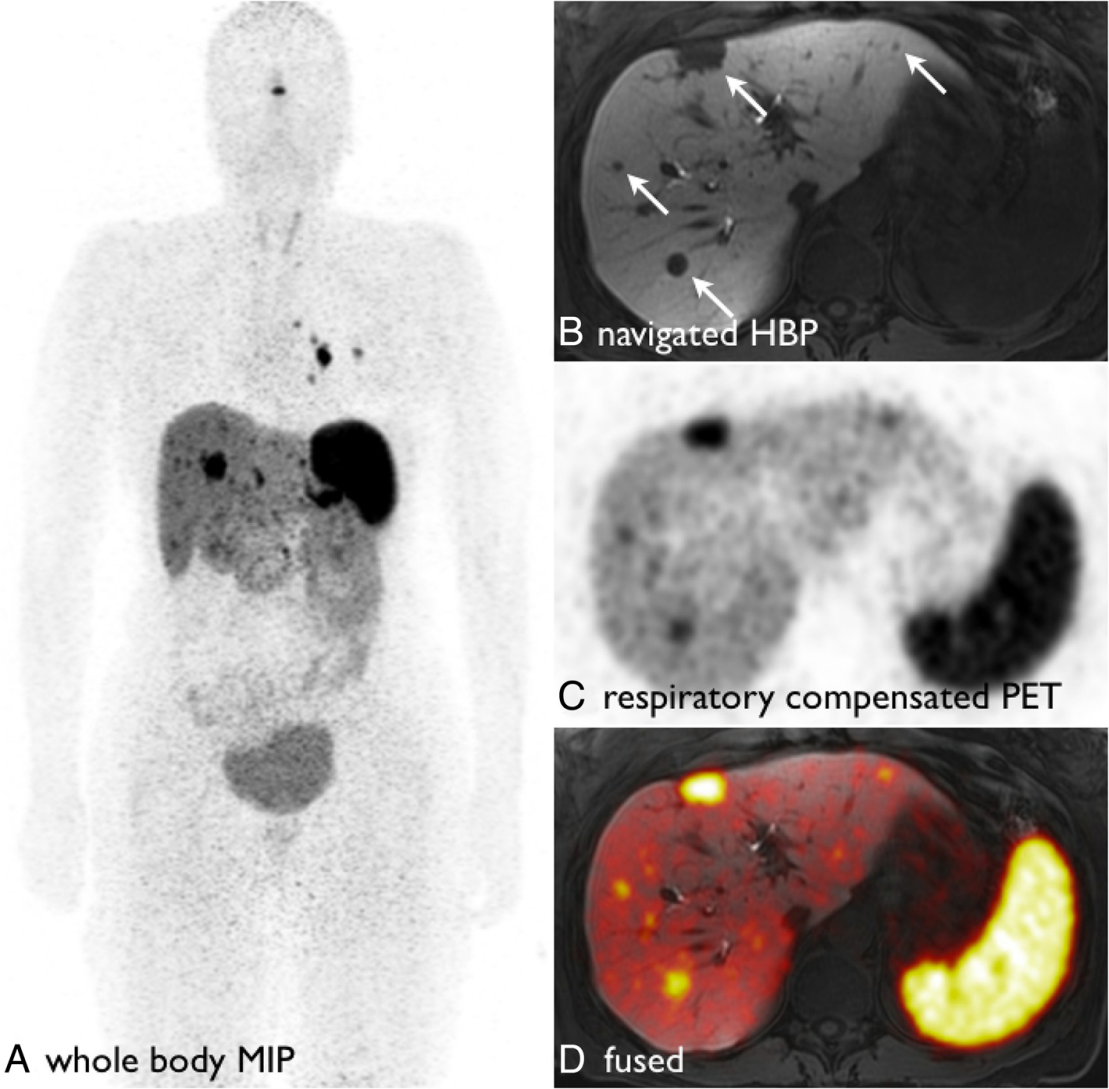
A 57-year-old female with metastatic neuroendocrine tumor to the liver, the mediastinum, and lung (a). Hepatobiliary phase imaging of the liver demonstrates multiple hypointensities (b, *arrows*) that correlate with DOTA-TOC uptake on respiratory compensated PET imaging (c). By combining the navigated HBP imaging and respiratory compensated PET data, accurate fusion between PET and MRI can be performed (d)
